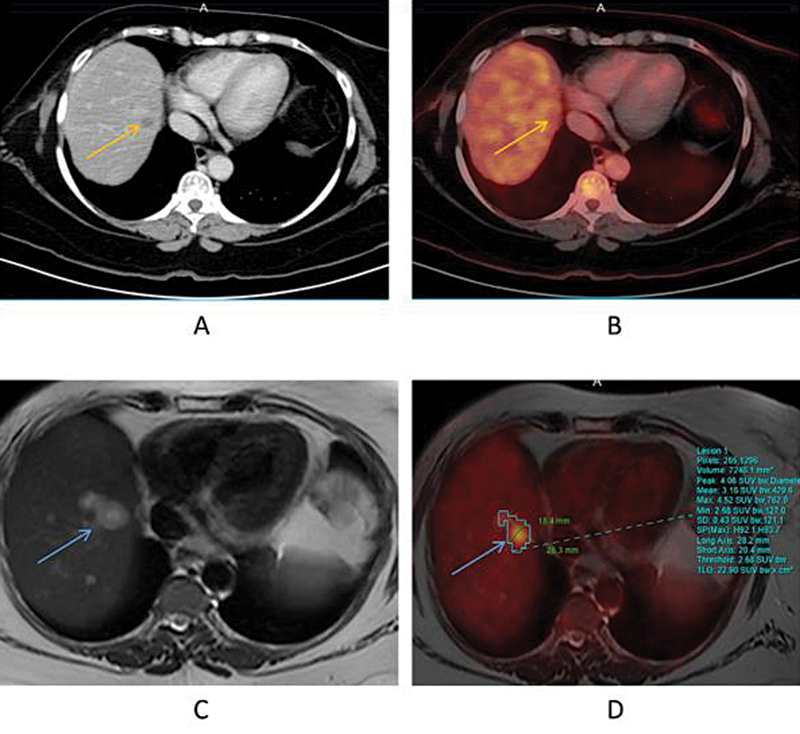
Case of breast cancer with liver metastases: Axial CT and simultaneous PET/CT images are shown in (
